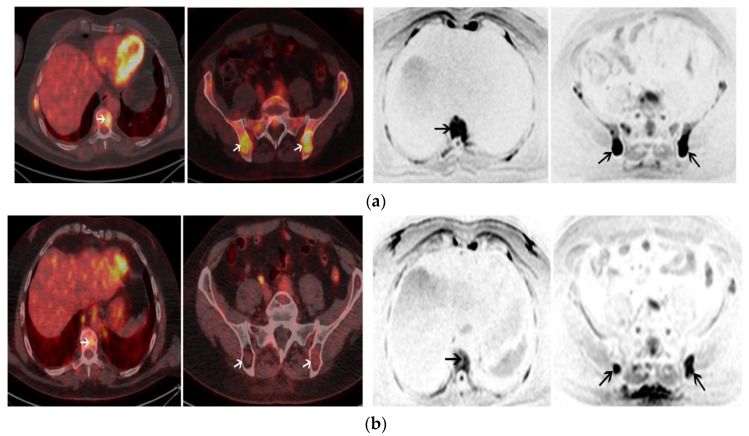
A 55-year-old male with IgA kappa MM. (a) Axial images of baseline 18-FDG PET/CT (left panel) shows multiple focal lesions (FL) with high uptake including one FL involving T9 and 2 FL involving the pelvis (white arrows). Axial images of baseline WB-DW-MRI (left panel) shows FL with restricted diffusion (b = 800 s/mm^2^) involving also T9 and the pelvic bone with an ADC of 920 (black arrows); (b) Positive post-ASCT 18-FDG PET/CT (left panel) shows a persistent uptake of T9 (FL SUVmax > liver uptake) and the regression of uptakes within FL involving the pelvis. Positive post-ASCT WB-DW-MRI (right panel) shows a regression of the restricted diffusion in T9 but the persistence of two FL in the pelvis with restricted diffusion and ≤25% increase of the ADC values (ADC = 1000). Patient was in complete remission after ASCT but relapsed 16 months after ASCT.
